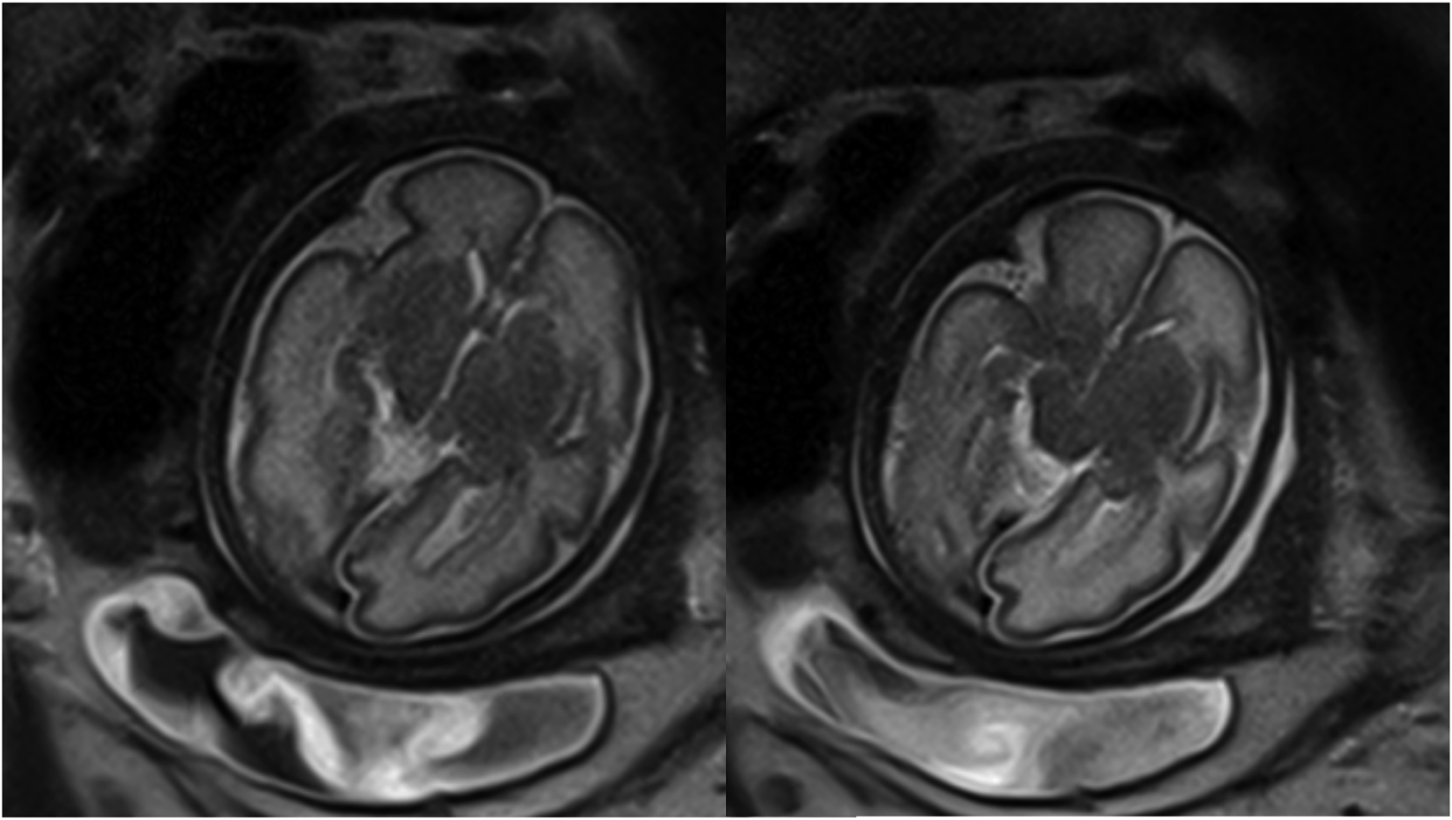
Fetal MRI at 33 + 2 weeks’ gestation. Symmetrical visualization of the cerebellar hemispheres with a sagittal extension of 3.5 cm (normal value 4.4 cm ± 3 mm). Formation of a vermis. Symmetrical presentation of the cerebral hemispheres, with gyral relief consistent with the 29th week of gestation. The biparietal diameter measures 7.4 cm (normal value 8.5 cm ± 3.5 mm), the cerebral biparietal diameter measures 6.6 cm (normal value 7.9 cm ± 3.9 mm). Fronto-occipital diameter is 8.5 cm (normal value 9.9 cm ± 3.7 mm). Signs of microcephaly with non-age-appropriate gyration (lissencephalic pattern). The development of the neurocranium as well as the calvarium corresponds to the 29th week of gestation.
